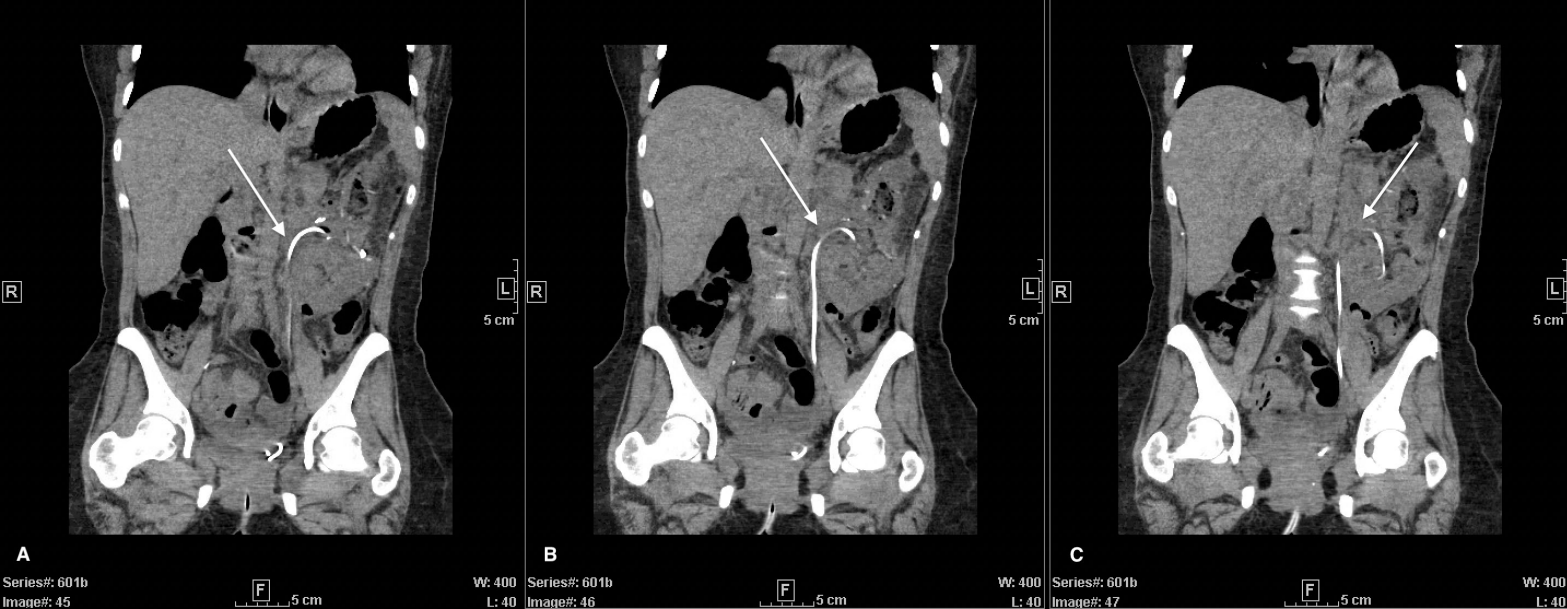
Post left partial nephrectomy CT-abdomen with IV contrast. Coronal view of 3 continuous images from left to right (A–C). Ureteral stent *(white arrows)* displaying continued arching trajectory superiorly despite removal of mass.
